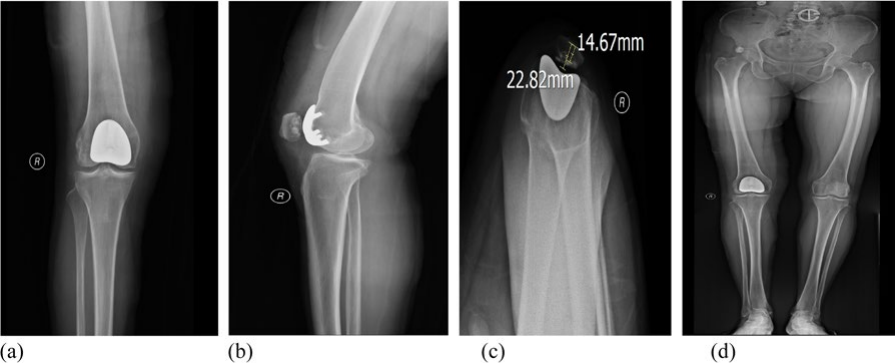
a Postoperative anteroposterior plain film of the knee joint (weight-bearing position), b Postoperative lateral plain film of the knee joint (weight-bearing position), c Postoperative axial flat patellar film (weight-bearing position), d Postoperative total-length plain film of the lower extremity (weight-bearing position)
